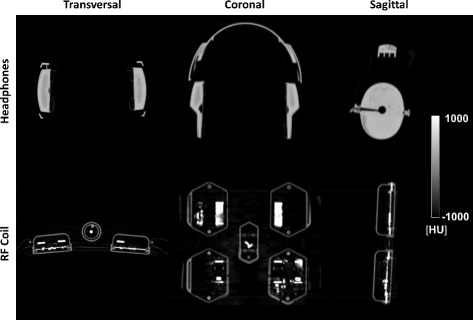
CT images of headphones and RF surface coil. The headphones are composed of two earpads connected with a flexible headband. The RF coil contains highly attenuating materials with CT values larger than 3071 HU, mandating the use of an extended CT scale. CT grayscale windowing: *C*=0 HU,*W*=2000 HU
